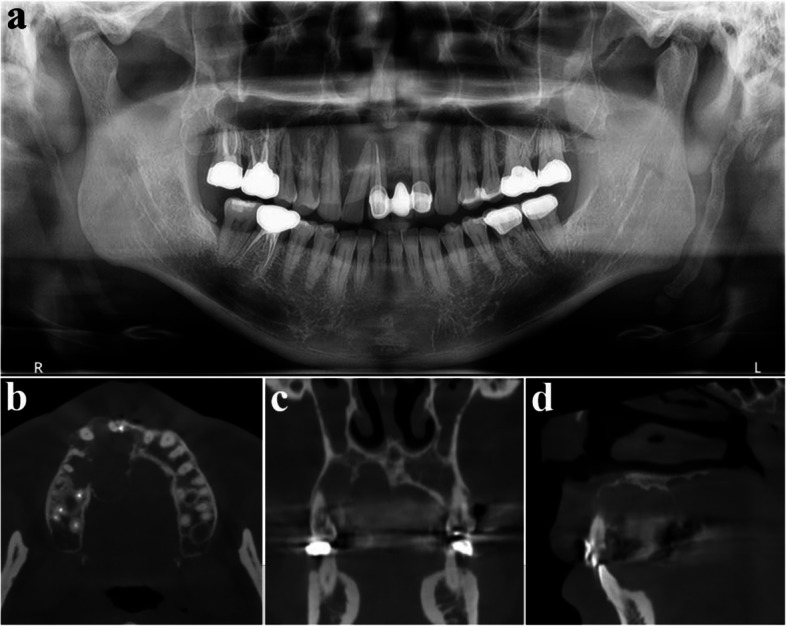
Preoperative radiographic images of a 41-year-old male patient with ameloblastoma in the maxillary anterior region, (a) panoramic radiograph, (b) CBCT, axial view, (c) CBCT, coronal view, (d) CBCT, sagittal view
